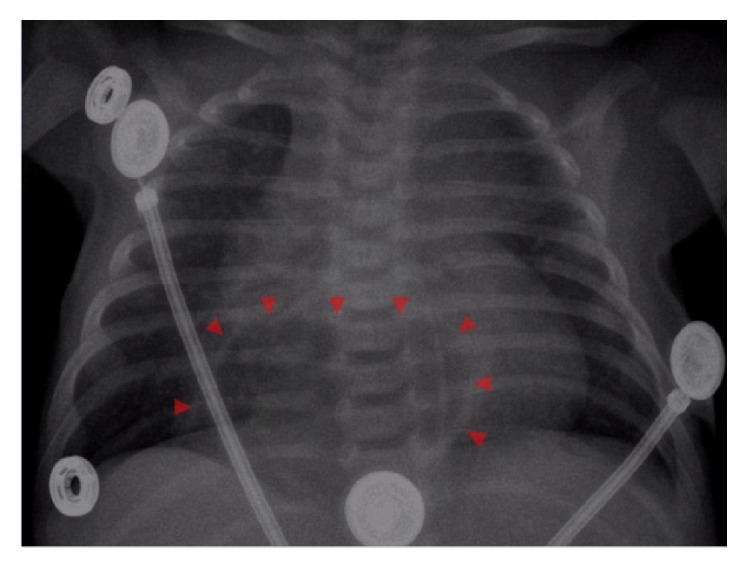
AP chest X-ray showing a radiolucency over the cardiac silhouette just above the diaphragm (arrowhead).
